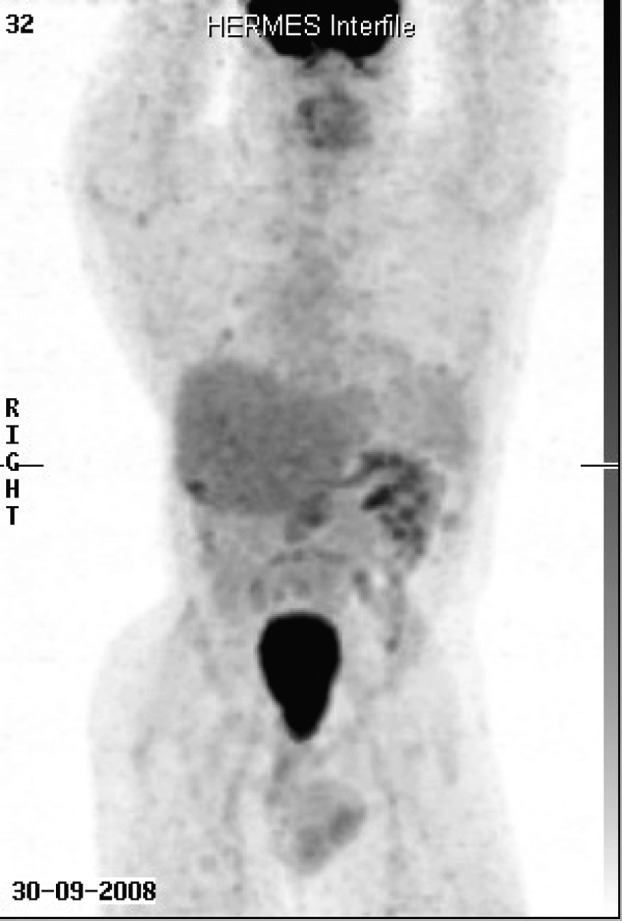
Staging positron emission tomography-CT scan showed uptake in the para-aortic region, the liver, a nodule inferior to the spleen, a nodule anterior to the proximal right latissimus dorsi muscle and variable uptake in the pulmonary nodules.
